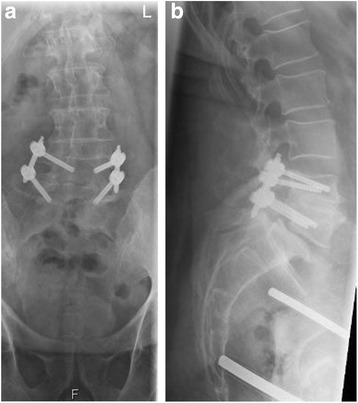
a and b Follow-up radiograph of the lumbar spine anterior-posterior and lateral at 11 months on 7 Dec 2015: unchanged position of the posterior instrumentation. Bony fusion of L4 and L5
